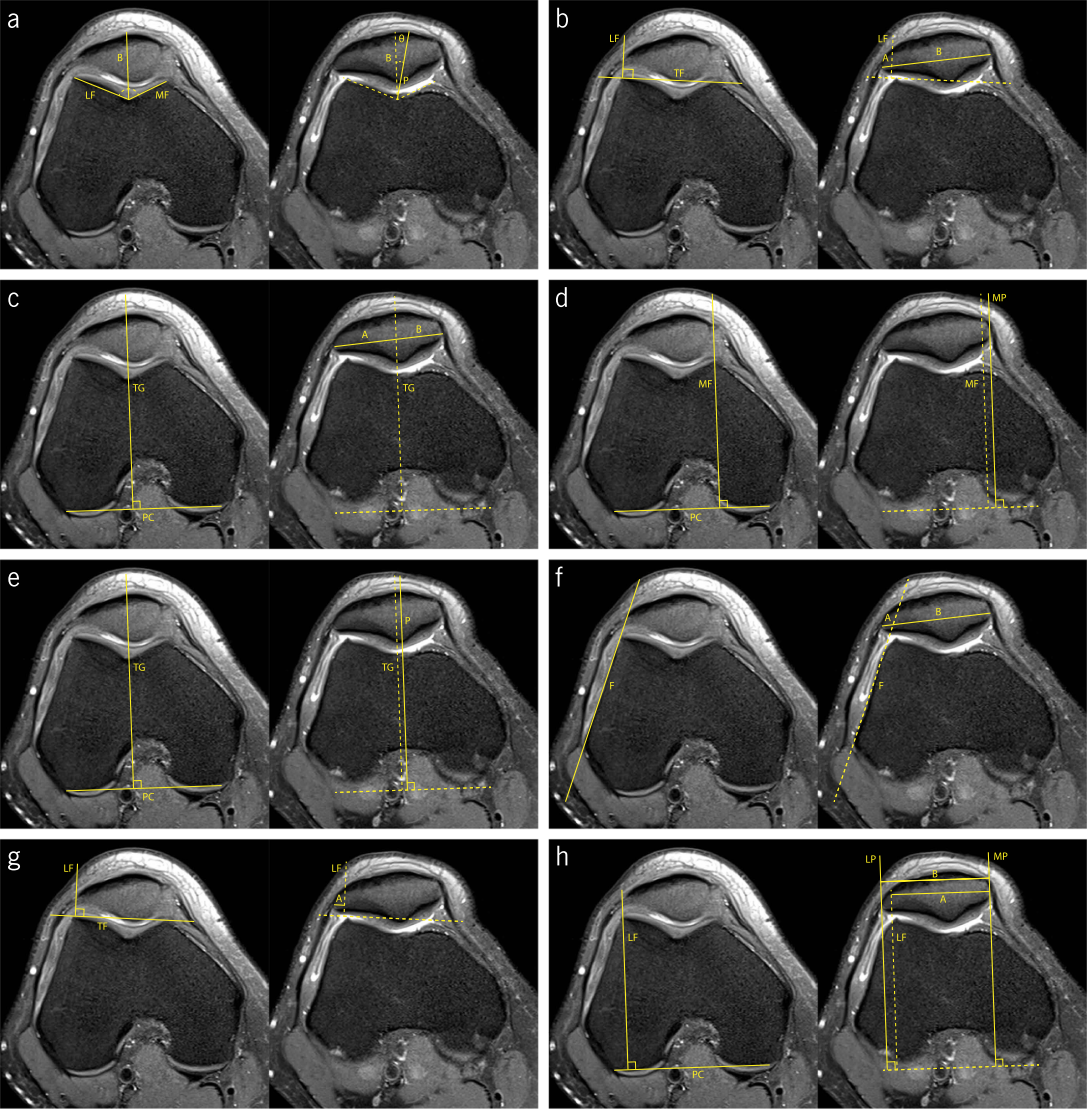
Fat-saturated proton density-weighted magnetic resonance images demonstrating the measurements of the indices to assess patellar lateralization. a Congruence angle (∠θ). b Patella–lateral condyle [A/(A + B)] and lateral shift (A/B). c Bisect offset ratio [A/(A + B)]. d Lateral patellar displacement (distance MF–MP). e Patellar displacement (distance TG–P). f Lateral patellofemoral length (length A) and tangent offset (A/(A + B)). g Lateral patellar edge (length A). h Patellofemoral axial engagement index (A/B)
